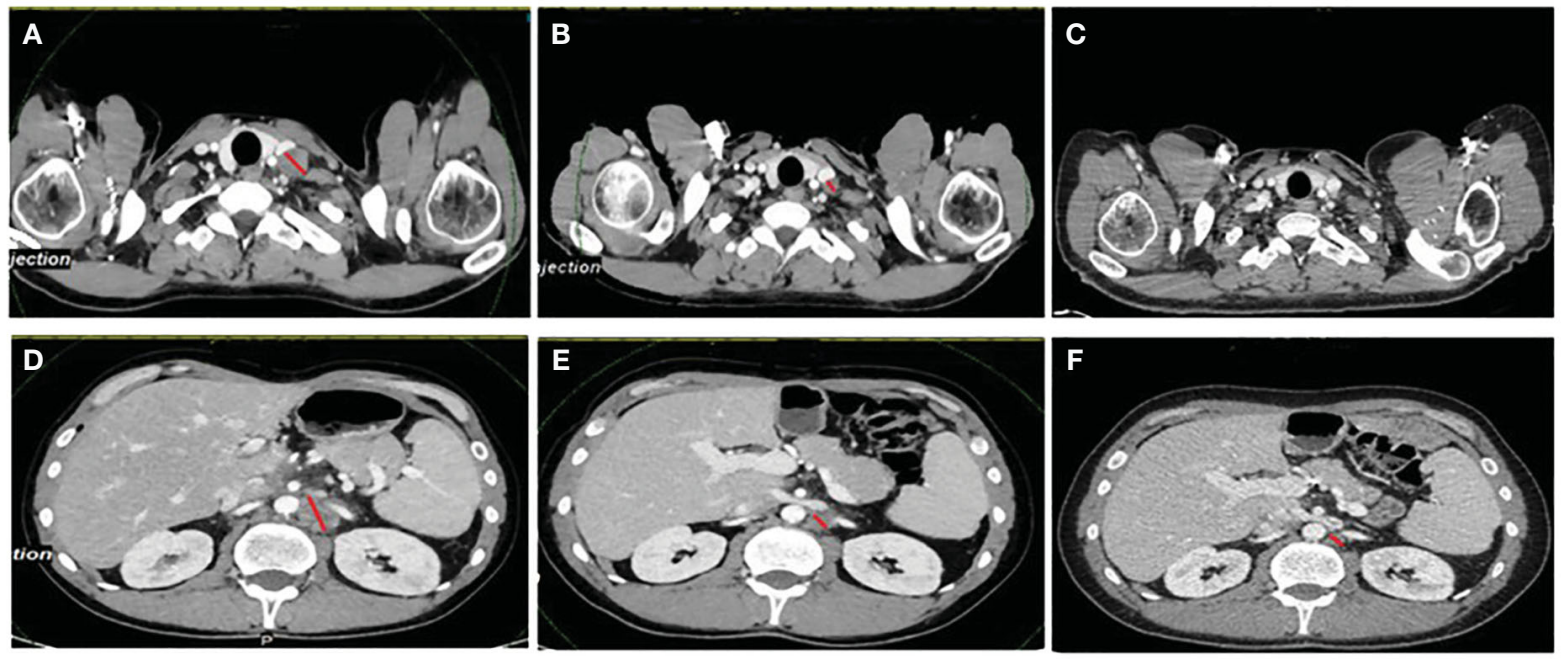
The patient’s CT scans at baseline, at best response, and at the last evaluation. (A–C) Evolution of a left supraventricular node. (D–F) Retroperitoneal node. The images in (A) and (D) were taken before starting immunotherapy treatment, while the images in (B) and (E) are those at the best response (this patient experienced a partial response with a −76% reduction in the target lesions). The images in (C) and (F) are the last scans of the patient in January 2024, when the decision to stop the treatment was made after 34 months. The red lines denote measurable disease.
